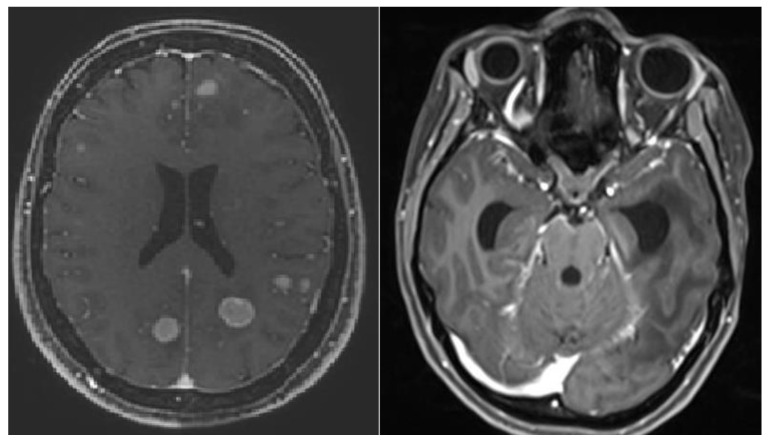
Representative MRI of diffuse distant brain failure: progression with more than 4 new lesions (left) and meningeal carcinomatosis (right).
